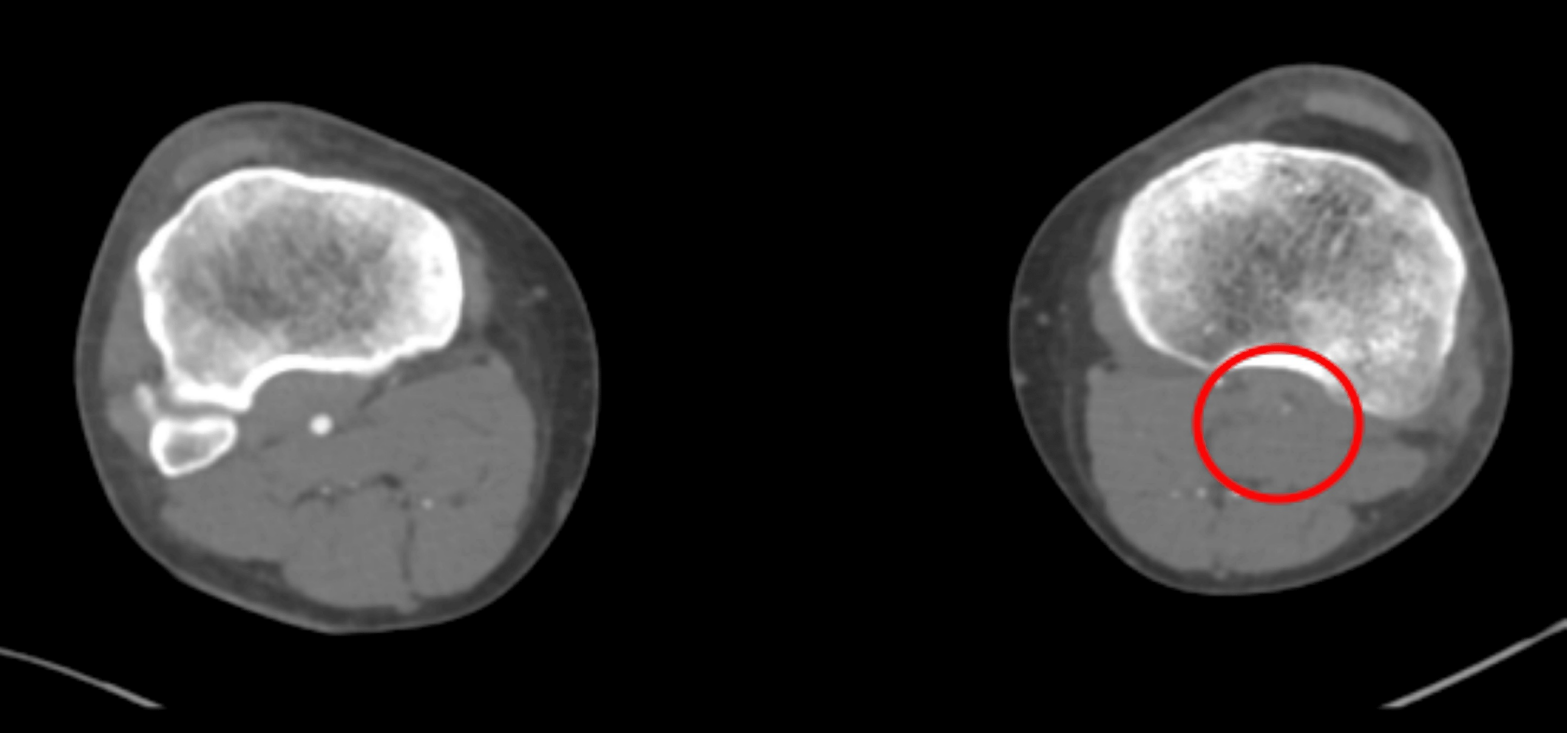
Initial CT angiogram showing left popliteal occlusion CT: computed tomography
